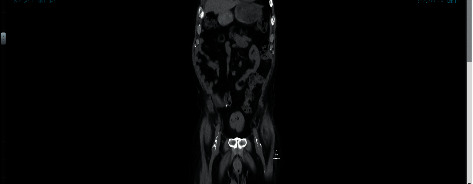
CT scan image demonstrating emphysematous cystitis with no demonstration of bladder perforation.
